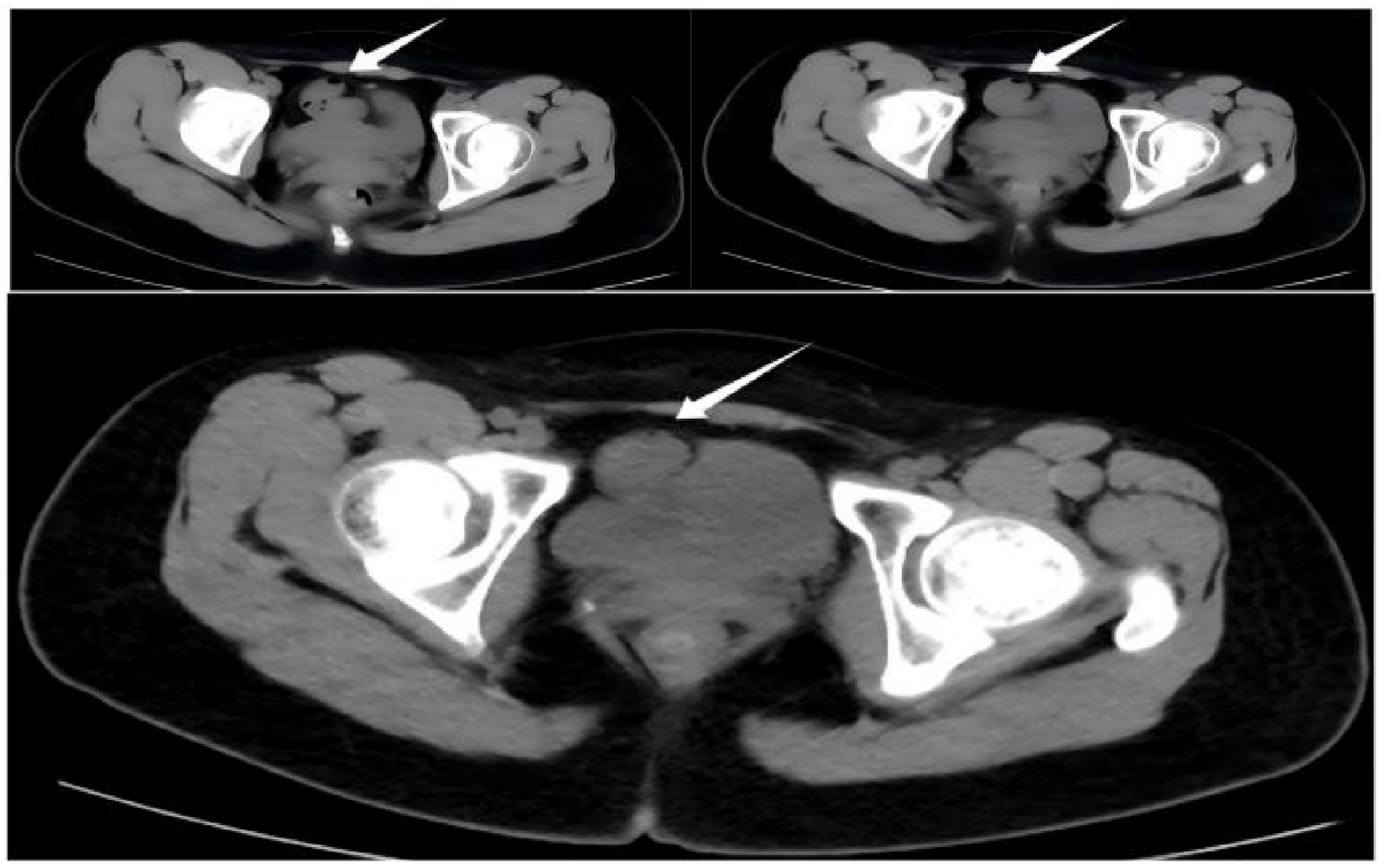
Non-contrast multi-slice CT images of the patient’s entire abdomen. A slightly increased density within the small bowel lumen in the pelvic region is visible, indicated by the white arrow.
